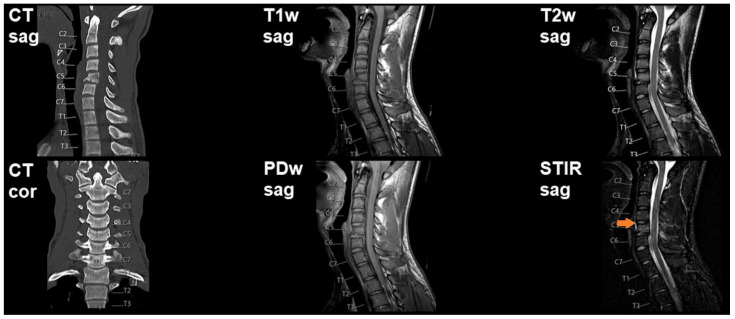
Preoperative imaging shows a fracture of the vertebral body of C5 according to computed tomography (CT). Cervical magnetic resonance imaging (MRI), using T1-weighted, T2-weighted, proton-density (PD)-weighted, and short tau inversion recovery (STIR) sequences, is suggestive of a subtle prevertebral hematoma (*orange arrow* in STIR imaging), but does not clearly reveal a ligamentous injury.
